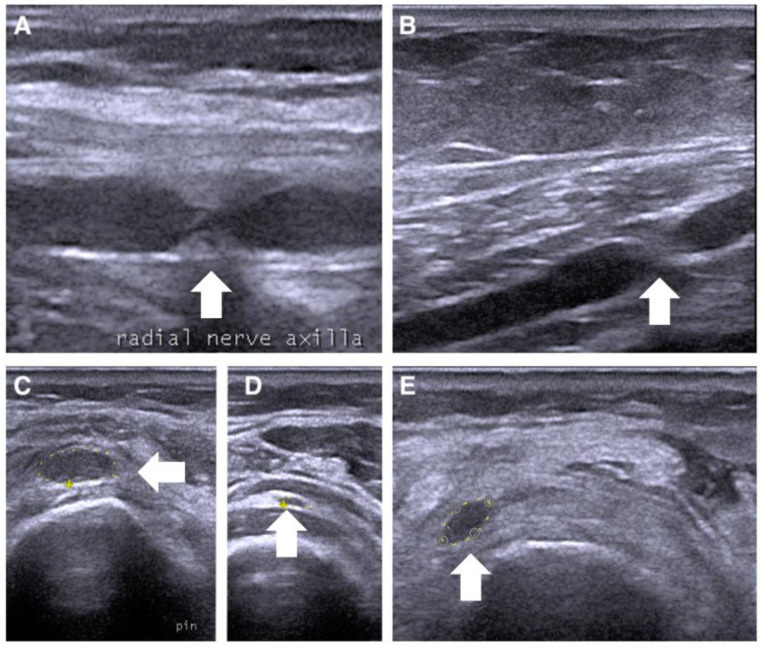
Ultrasonographic appearance of the second case of radial nerve torsion (white arrow) in at the upper arm in (A,B) on short axis, and the posterior interosseous nerve between the heads of supinator muscle (C–E) on long axis.
